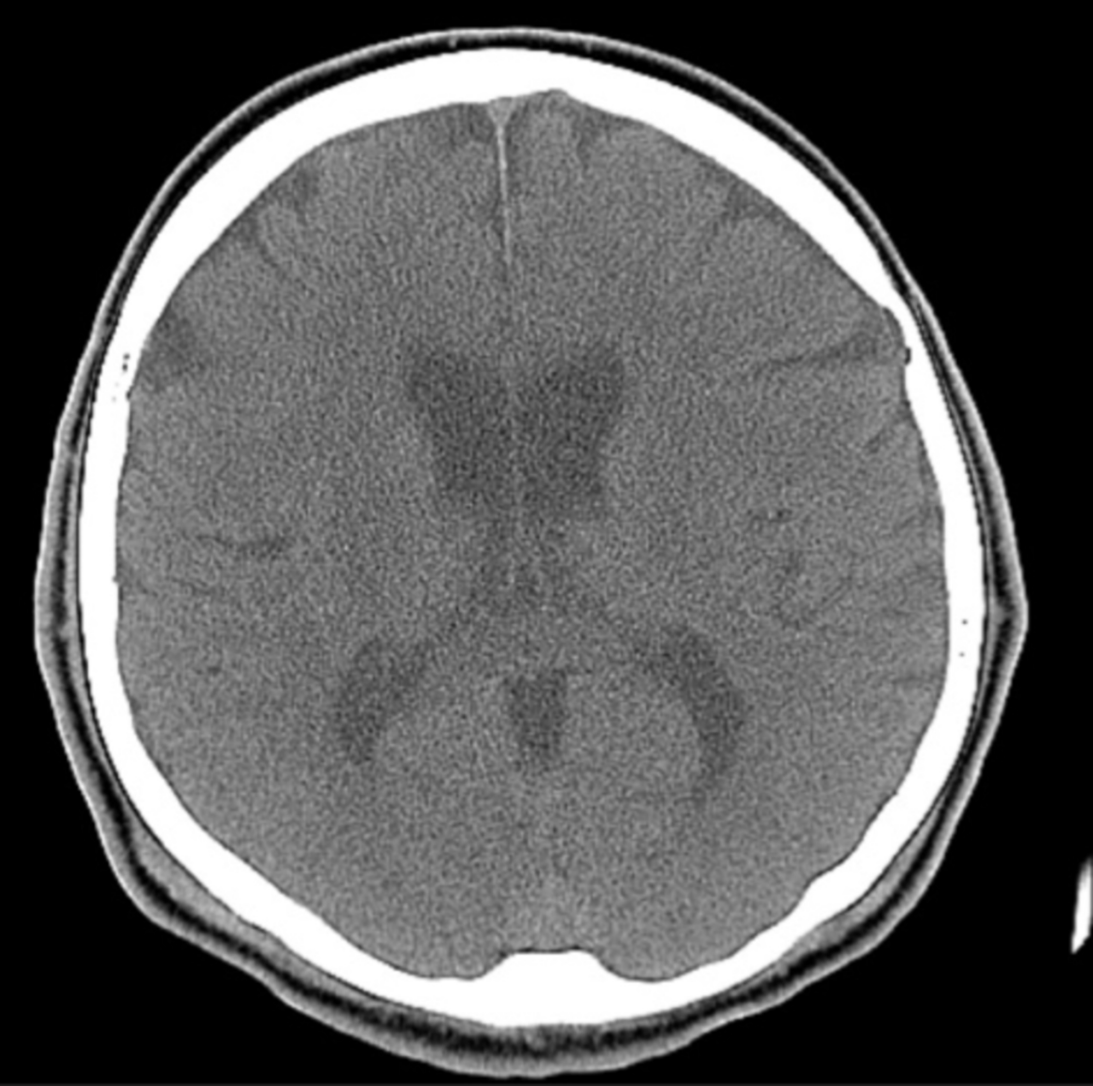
CT of the head No acute ischemic or hemorrhagic infarcts, midline shift, hydrocephalus, herniation, or old infarcts. CT: computed tomography
